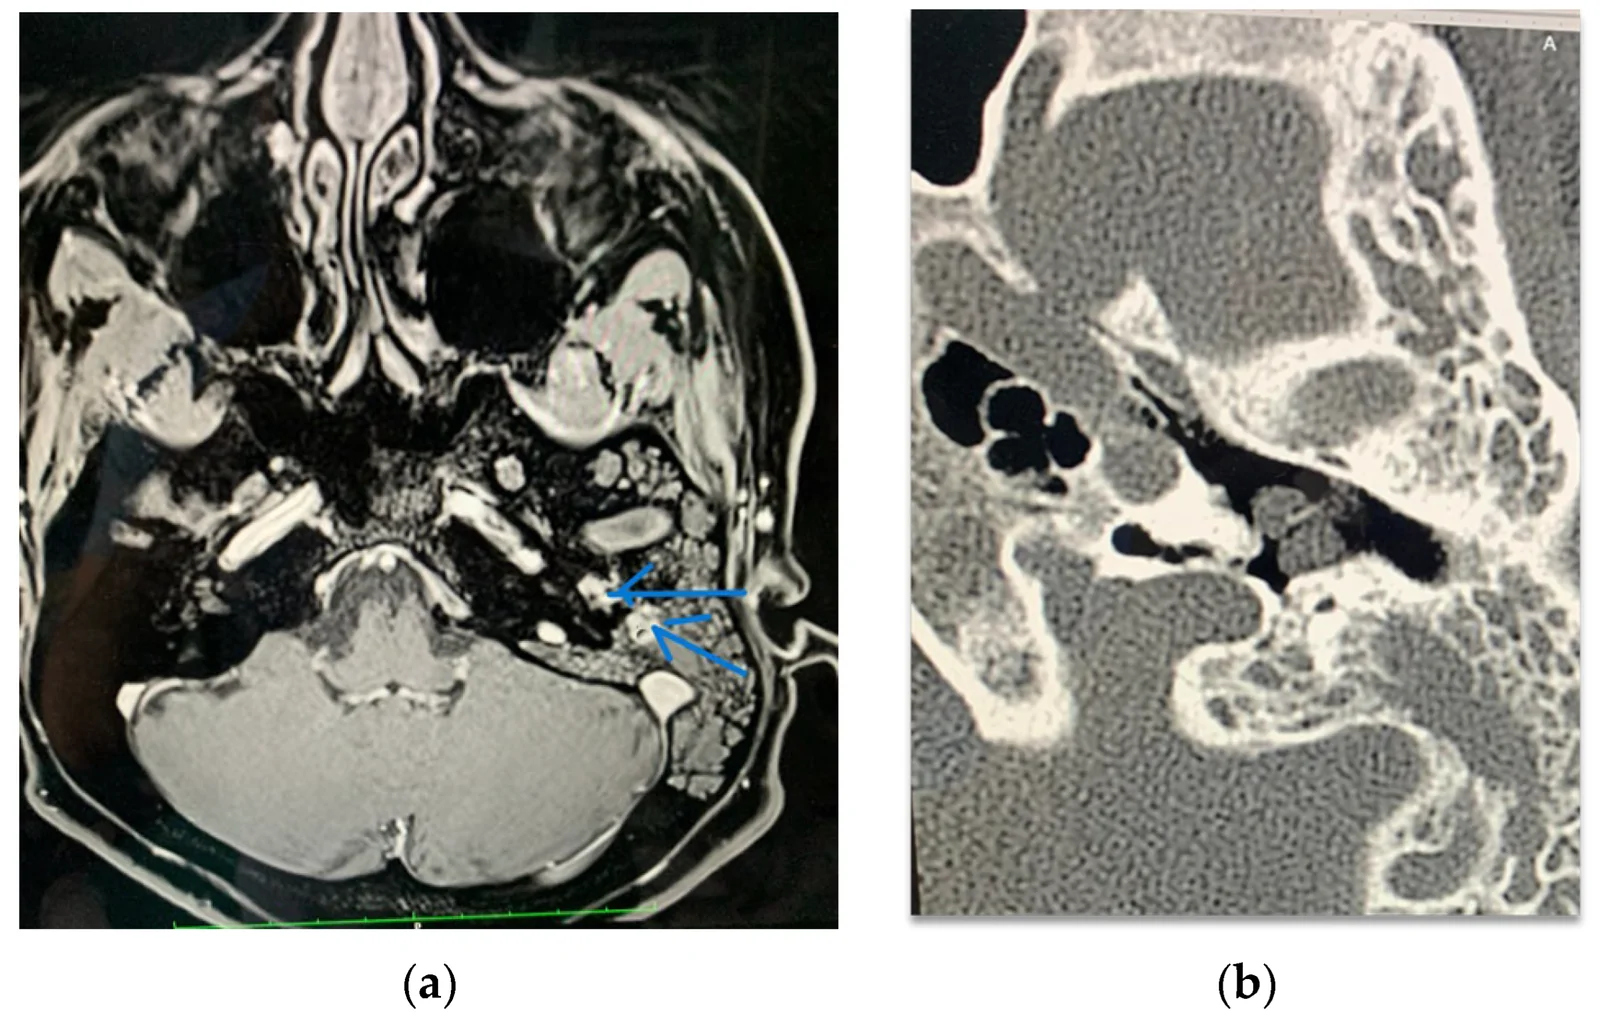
Imaging studies performed on Patient 1 (A.P.): (a) MRI scan—the arrows show a focal lesion in the left middle ear; (b) The CT scan of the left ear.
